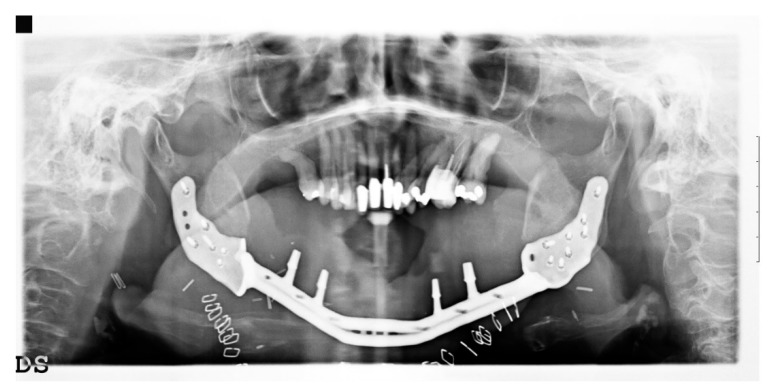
Orthopantomography two months after surgery.
